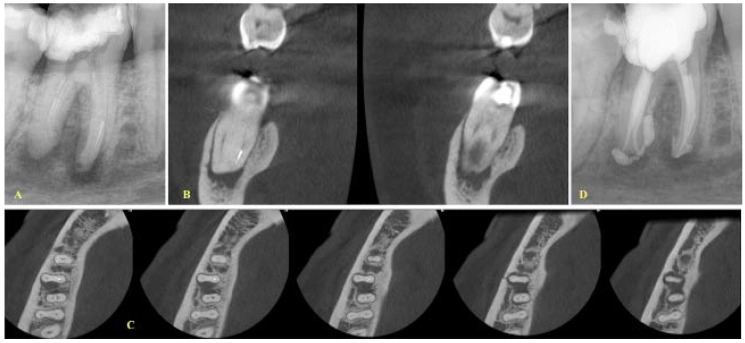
(A) Initial radiograph (mesial angle view) of a mandibular first molar with periapical lesions and a fragment at the apical third of the mesial root. (B,C). Coronal and axial views demonstrating that the two canals are separate, and the fragment is located in the mesiolingual canal, (D) A treatment plan decision to bypass the fragment was made due to the presence of a large lesion, especially at the lingual aspect of the cortical bone. Final radiograph of the case where the bypass of the fragment is evident.
